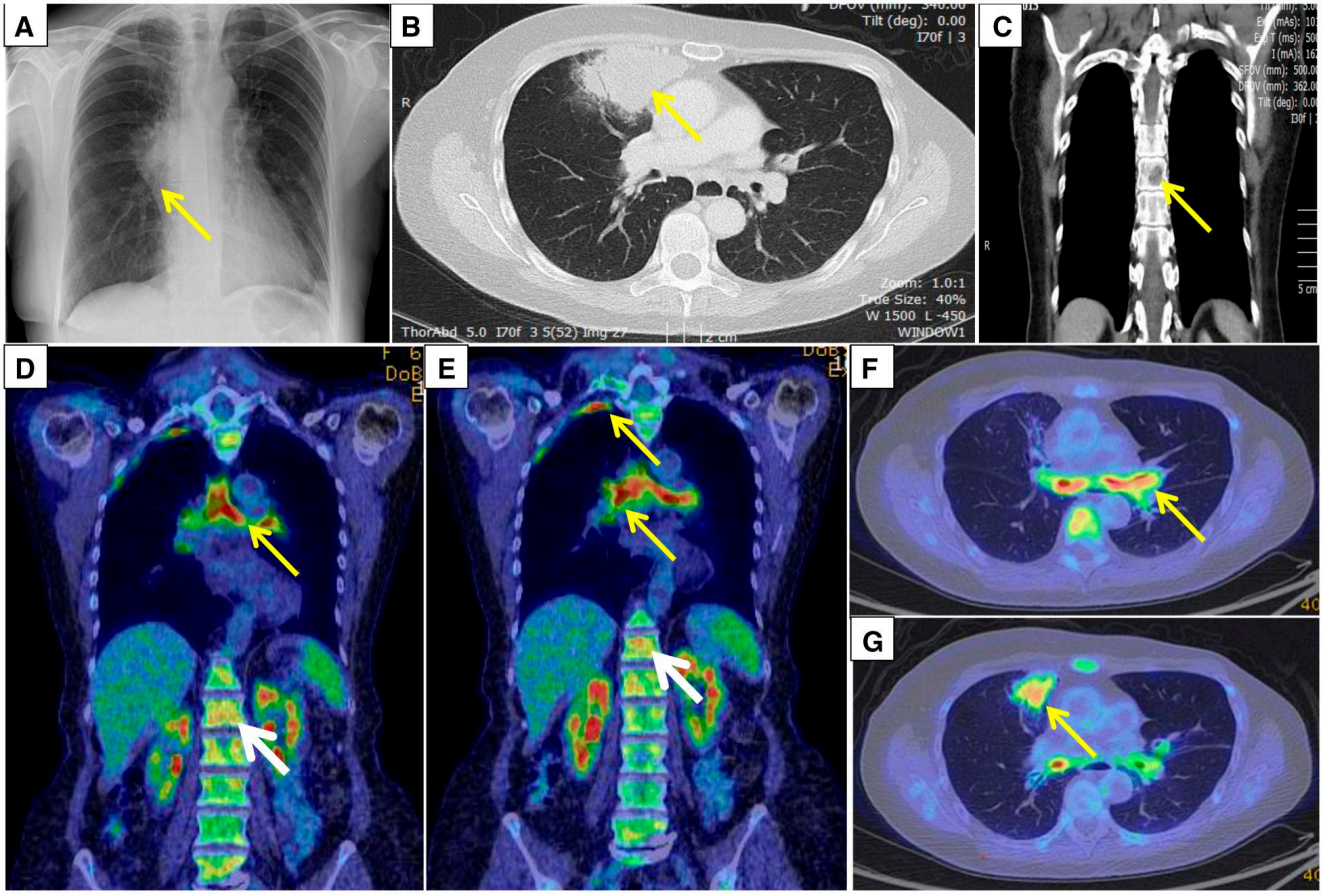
Chest X-ray, CT thorax and CT-PET imaging. (A) Chest X-ray, showing new right hila shadowing. (B) CT thorax image, showing right middle lobe mass with air bronchogram. (C) Multiple lytic lesions in the spine, suggesting bony metastases. (D–F) [^18^F]Fluorodeoxyglucose-avid trachea and bronchi and inferior pleural surface (yellow arrows). (D, E) Reactive homogeneous increase in marrow uptake (white arrows). (G) Wedge-shaped consolidation in the right middle lobe
